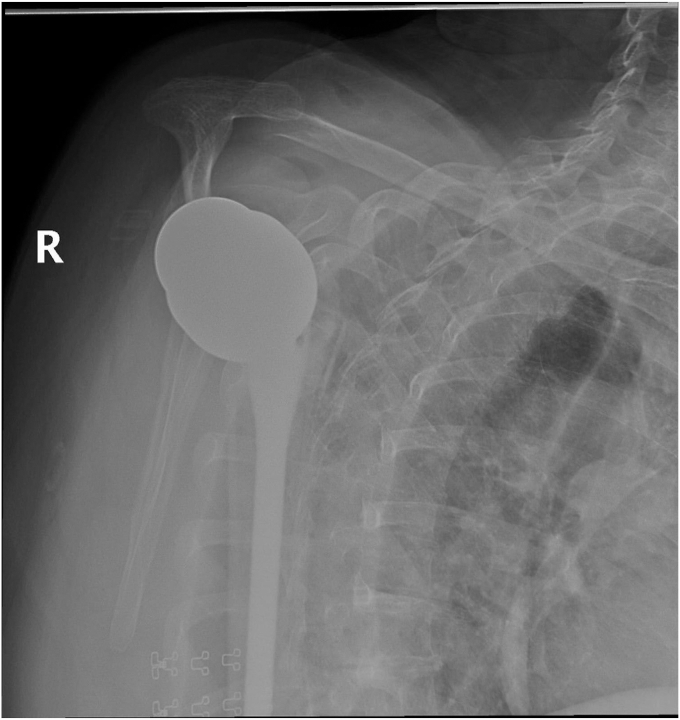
2-week postoperative radiographs following distal clavicle resection for large central glenoid defect and adequate glenoid baseplate fixation.
